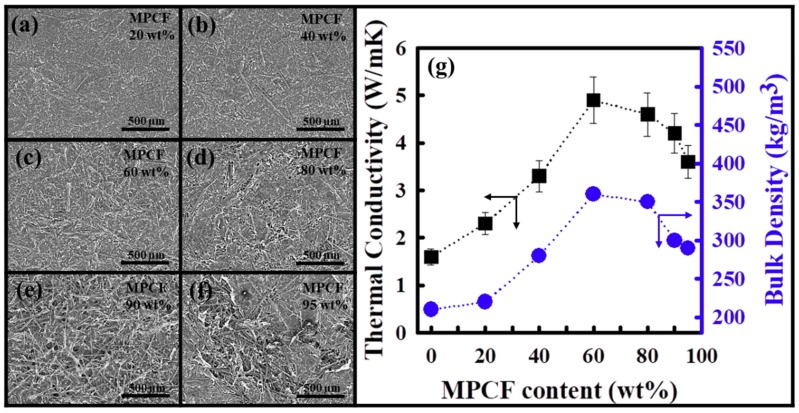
HR-SEM images of the FWCNT-MPCF hybrid films with different mesophase pitch-based carbon fiber (MPCF) (length: 200 μm) contents: (a) 20 wt %, (b) 40 wt %, (c) 60 wt %, (d) 80 wt %, (e) 90 wt %, and (f) 95 wt %; (g) thermal conductivity (black quadrangles and arrows) and film bulk density (blue circles and arrows) of the FWCNT-MPCF hybrid films with different MPCF (length: 200 μm) contents. These FWCNT-MPCF films were prepared by vacuum filtration following ultrasonication for 0.5 h.
